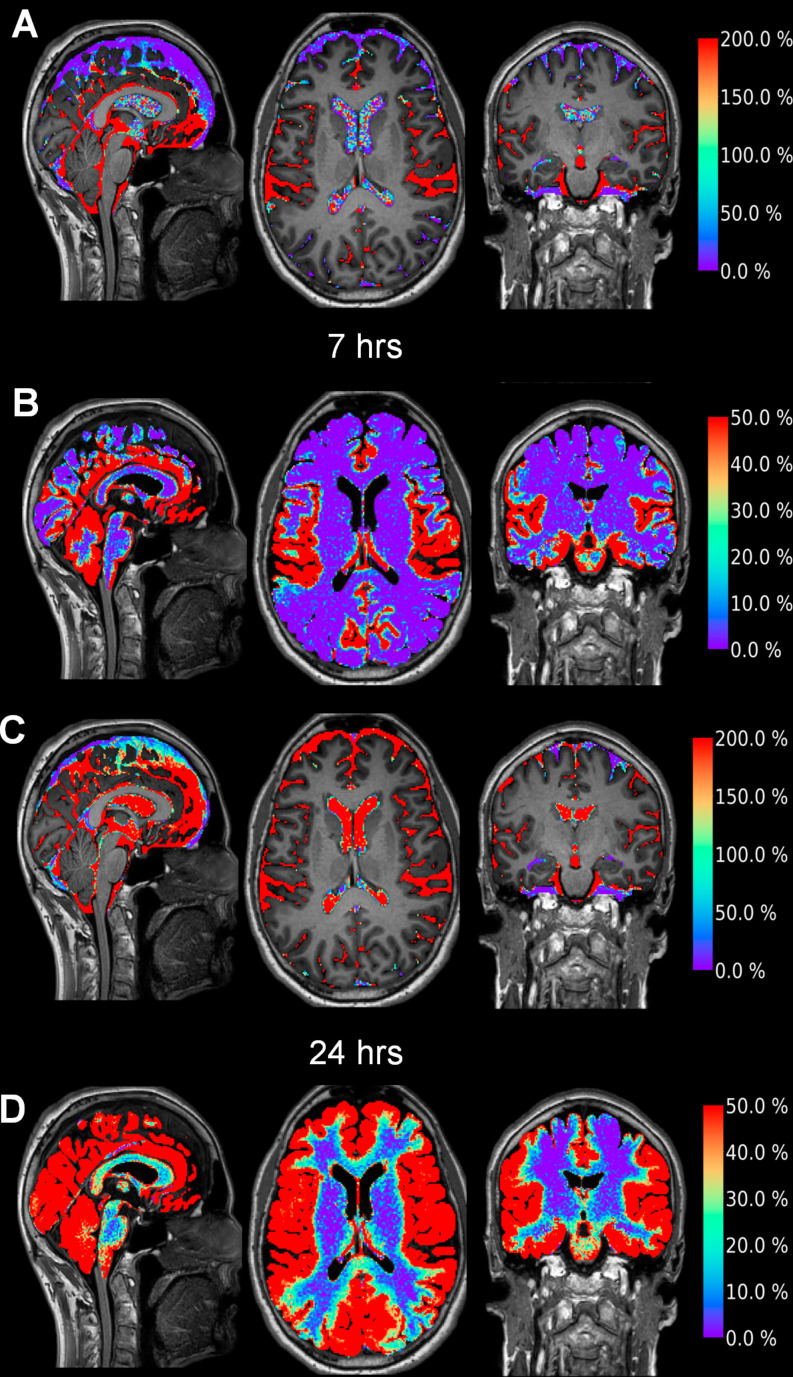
Glymphatic enrichment of a CSF tracer in a human subject is depicted in this figure. Currently, intrathecal contrast-enhanced MRI is considered the gold standard for glymphatic imaging in humans. Following the intrathecal injection of a CSF tracer, such as gadobutrol (Gadovist, Bayer, GE; 0.50 mmol, total volume 1 ml), tracer enrichment is visualized using standardized MRI T1 acquisitions, as previously described. The tracer first enriches the subarachnoid spaces (A), then progresses to the cerebral cortex and subcortical white matter (B), as indicated by the percentage increase on the color bars to the right at 7 h. It’s worth noting that the strongest enrichment within the subarachnoid space (A) corresponds to the area of strongest enrichment in the cerebral cortex (B). By 24 h, tracer enrichment remained comparable in the subarachnoid space but increased in the cerebral ventricles (C), while glymphatic enrichment became brain-wide at this time (D; percentage increase in tracer enrichment shown on the color bars to the right). The tracer gadobutrol is hydrophilic and does not pass the blood-brain barrier; instead, it remains confined to the extravascular compartment when administered intrathecally. It is a neutral compound with a molecular weight of 604 Da. Images provided by Lars Magnus Valnes, PhD, Department of Neurosurgery, Oslo University Hospital-Rikshospitalet
